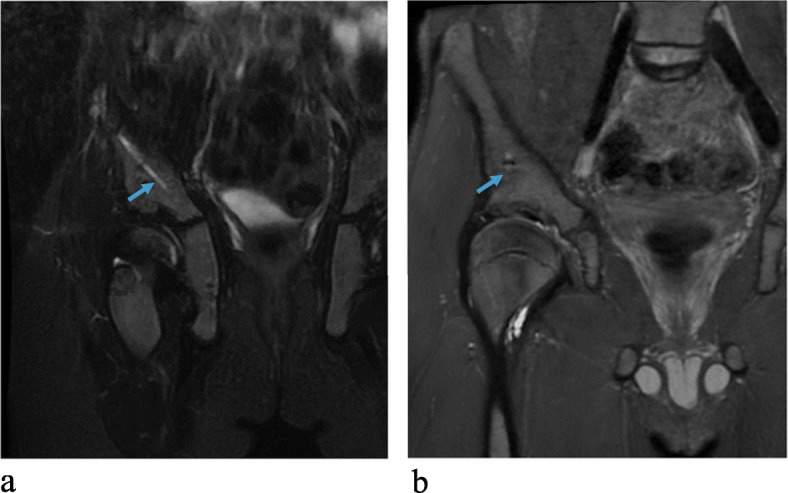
a, b. An MRI, performed 1.6 and 4.5 years postoperatively, respectively, for a patient with Perthe’s disease. Note in a (coronar T2W FS/1.5T ) that the screw canal is still clearly visible and filled with fluid (arrow) while in b ( coronar PD FS/3T), the screw canal is completely replaced with bone and a minimal metal artefact (arrow) can still be seen
